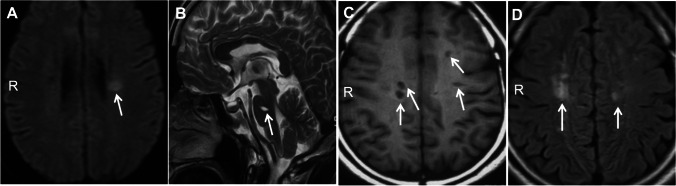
MRI brain of the index case’s brother (III9). MRI showed an acute infarct in the left corona radiata (a, DWI) and chronic infarcts in the pons (b, T2-weighted) and bilateral subcortical white matter (c, T1-weighted and d, FLAIR)
